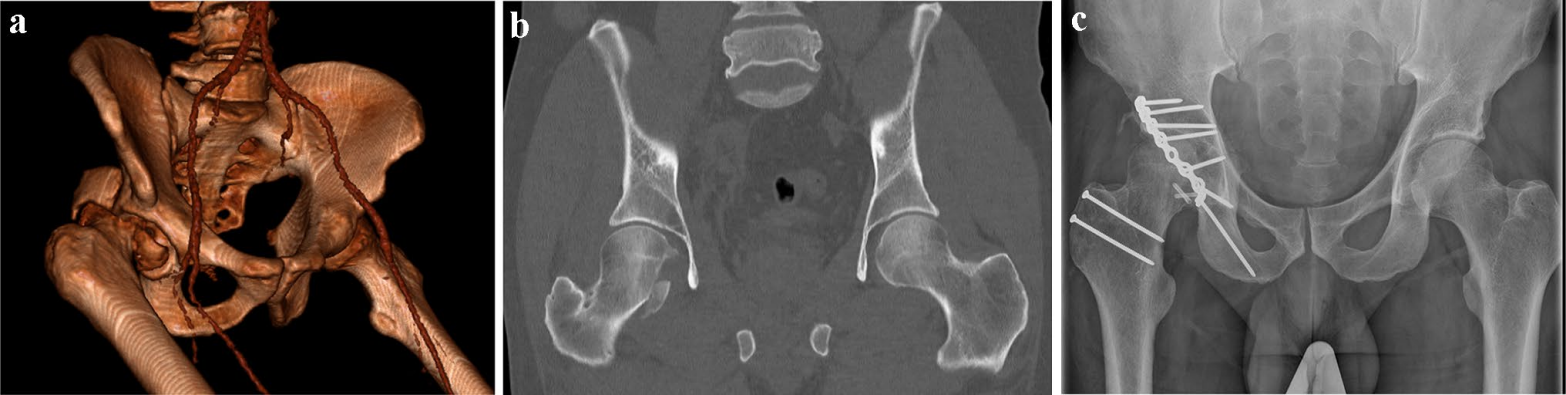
a 3D-CT scan before closed reduction of the hip joint in a 50 years old male after a traffic accident, displaying a Type 4 Pipkin fracture. b CT scan in the same patient after closed reduction of the hip joint displaying the fracture of the femoral head. c Postoperative x-ray in the same patient after plate fixation of the acetabular fracture and fixation of the femoral head fragment using headless screws
